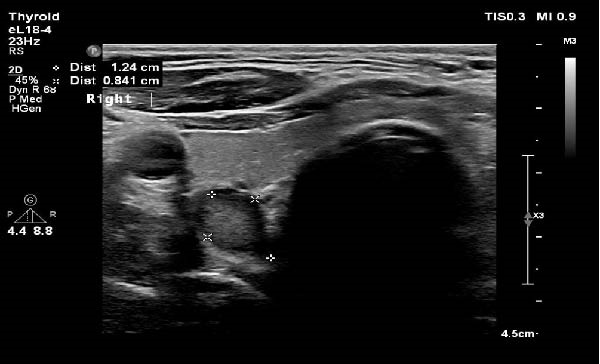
Parathyroid ultrasound demonstrates a well‐circumscribed, 1.2 cm lesion in the right inferior parathyroid region, consistent with a parathyroid adenoma.
